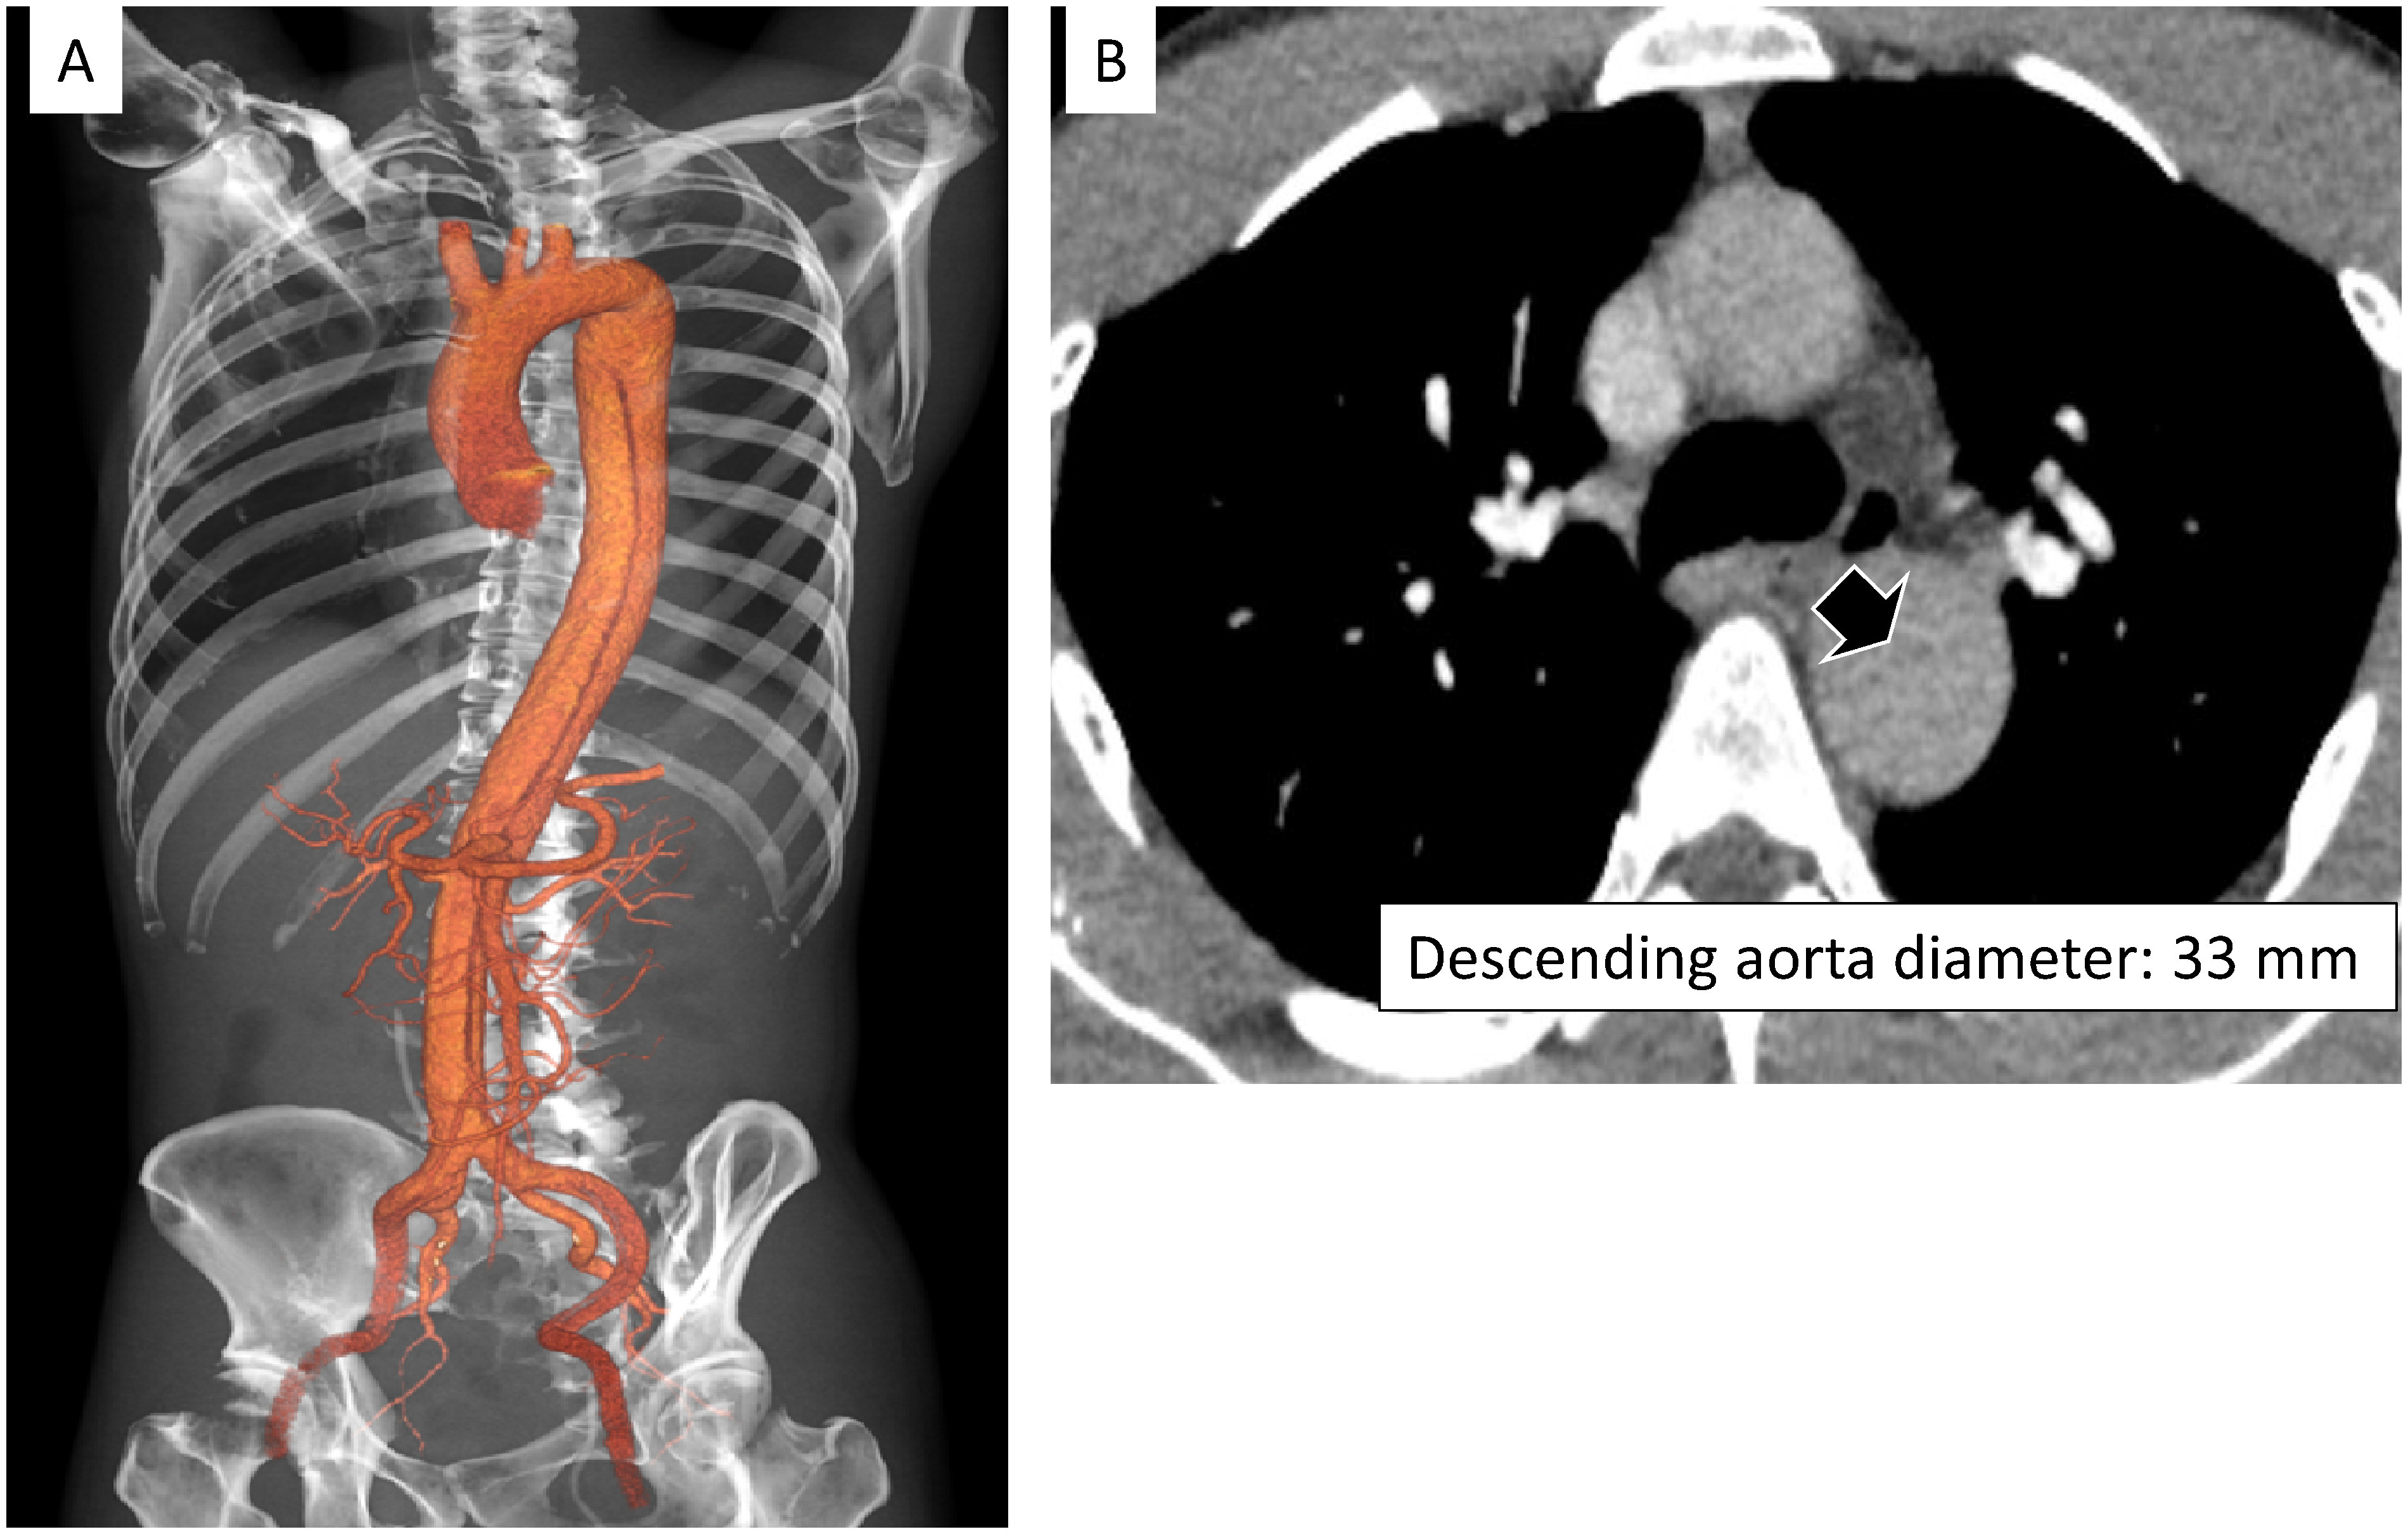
Fig. 1 (A) Three-dimensional computed tomography (CT) angiography on the day of onset. (B) CT on the day of onset of aortic dissection. Maximum axial diameter of the descending aorta, 33 mm. Arrow: flap.
